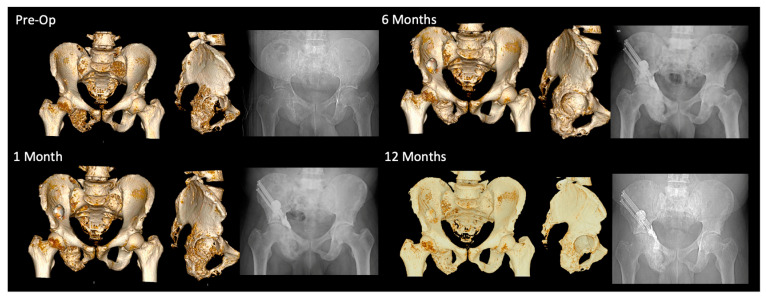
Plain X-ray radiograph and CT reconstruction view of periacetabular disease progression across 12 months. Patient experienced documented visceral organ progression with no history of radiation therapy or chemotherapy. Patient had history of bisphosphonate therapy during the post-operative period and was alive and ambulating well at time of study censure. Measured relative bone mass improvement of 33.7% on 3D volumetric grayscale and 2D coronal, axial, and sagittal of 21.1%, 63.4%, and 16.1%, respectively.
